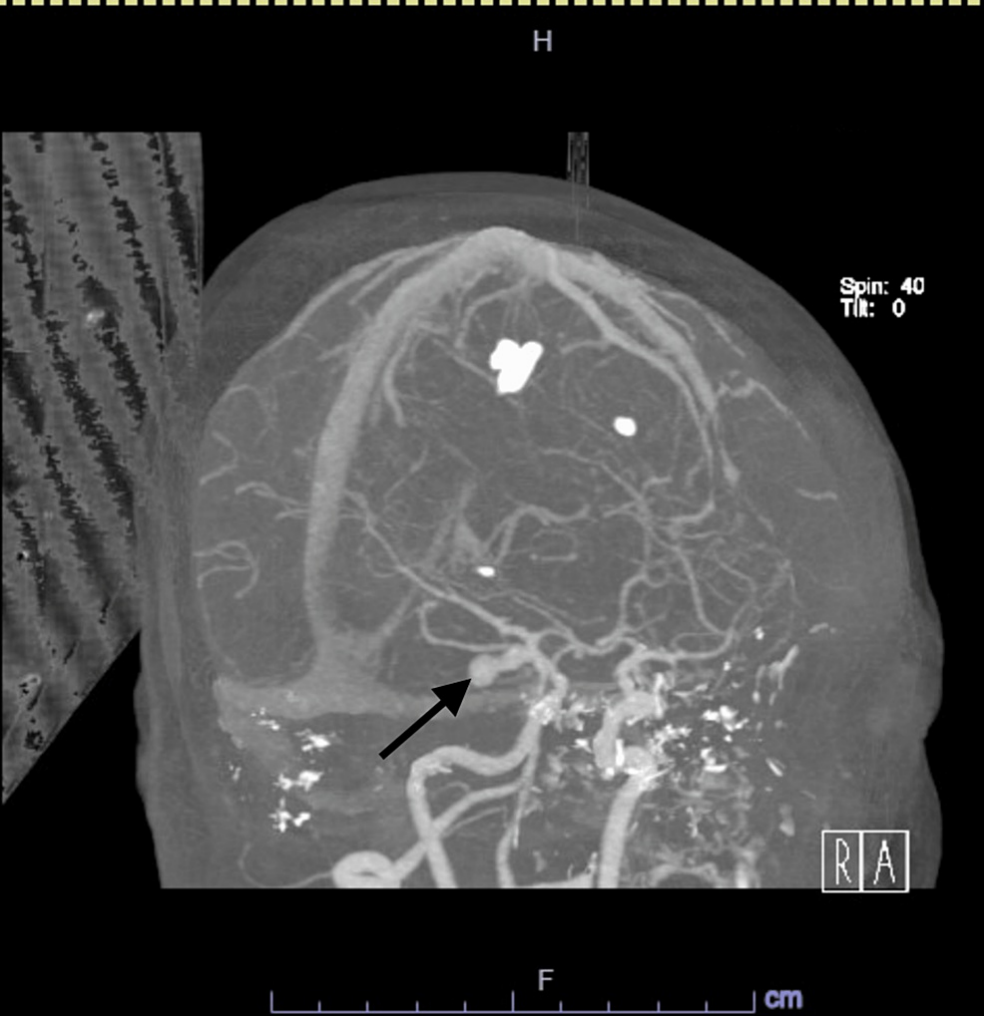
CTA Brain Computed tomography angiography (CTA) brain showing a multilobulated aneurysm formation affecting the right posterior communicating artery (arrow).
